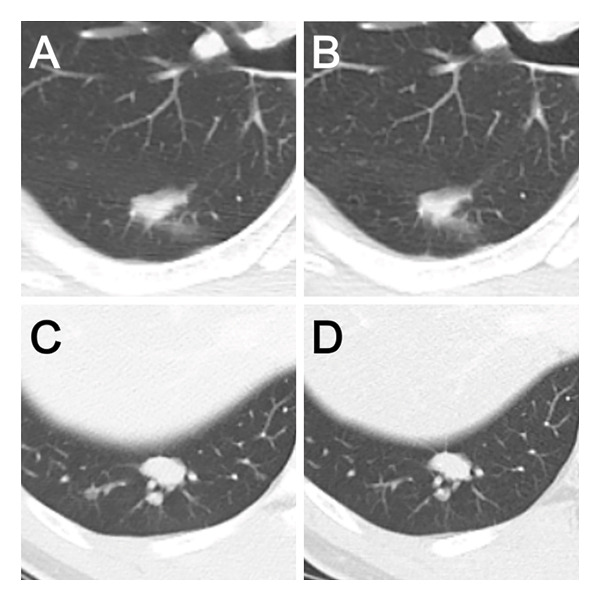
Clinical course of radiological findings. CT revealed two nodules in the right lower lobe at S6 (A) and S10 (C) before chemotherapy. The sizes of the two nodules remained unchanged after three courses of doxorubicin and ifosfamide (B) and (D).
